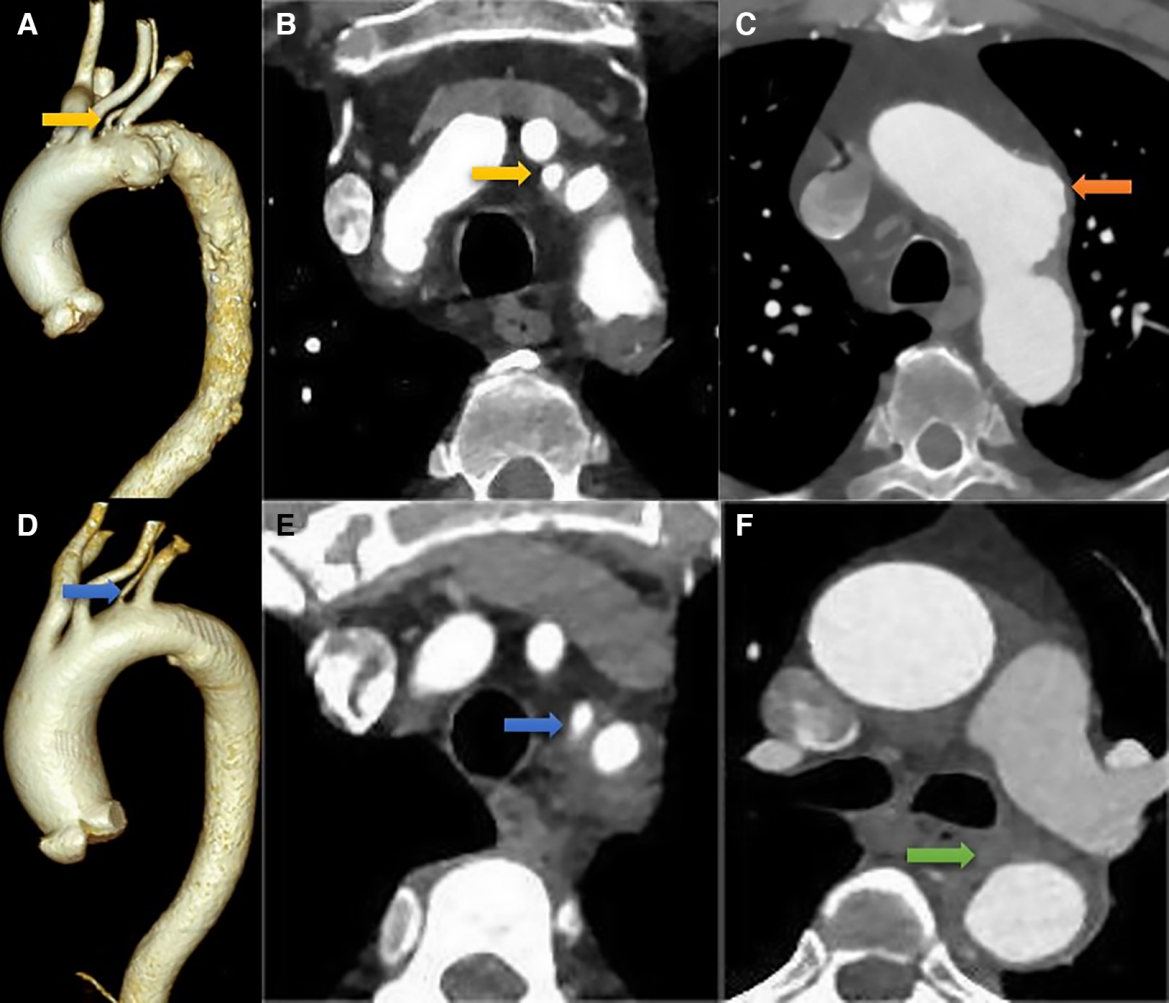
Preoperative CTA. (A–C) in a patient with thoracic aortic aneurysm, the ILVA (the yellow arrow) and aortic aneurysm (the orange arrow) are evident on the cross-sectional and three-dimensional reconstruction images; (D–F) in a patient with type B intramural hematoma, the ILVA (the blue arrow) and intramural hematoma (the green arrow) can be clearly seen from the cross-sectional and three-dimensional reconstruction images.
